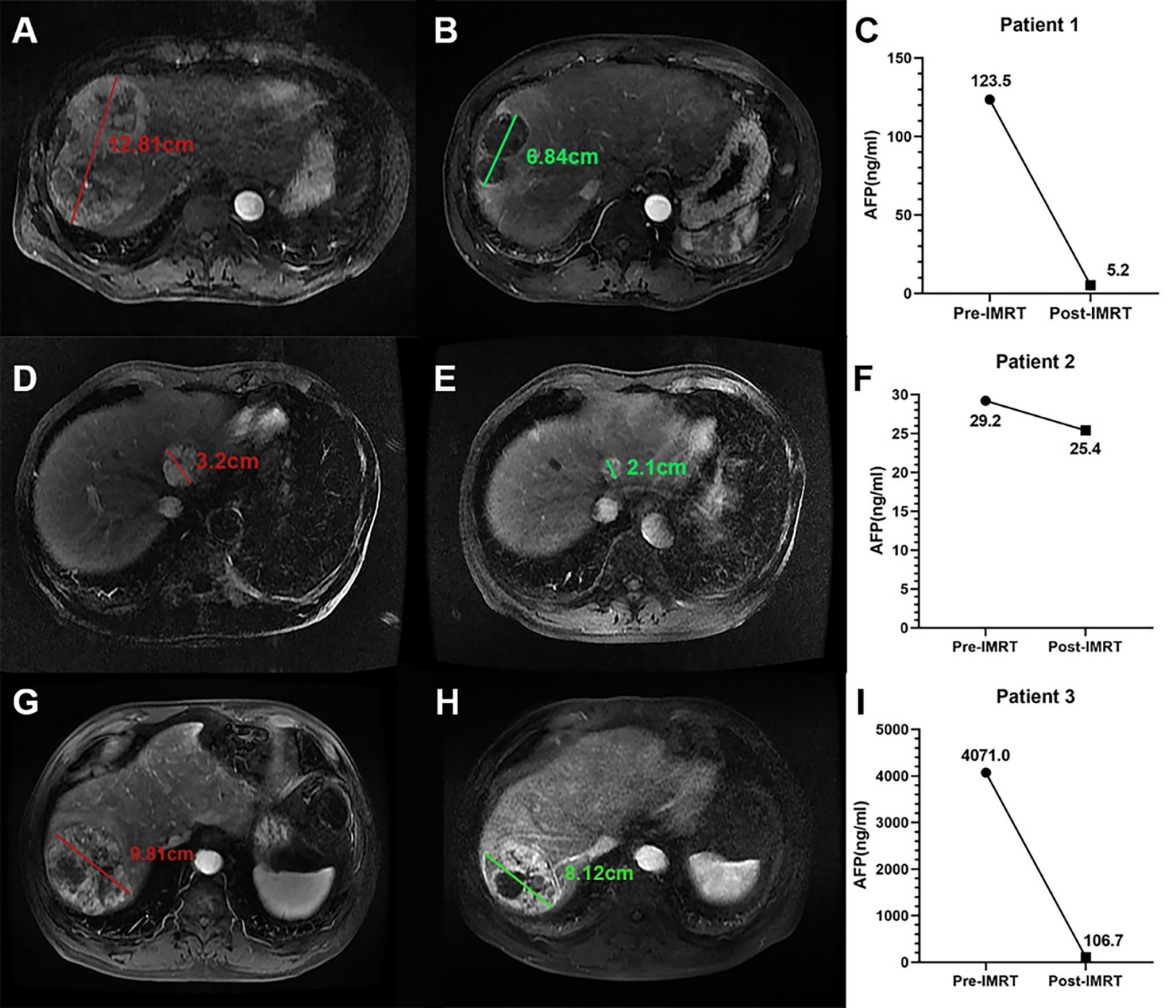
Three example cases of PR defined by the α-RECIST. (A, B) present the SLD on arterial phase images of pre- and post-IMRT CE-MRI scans for patient 1, and the size change is -42.0%; (C) presents the serum AFP levels before and after IMRT for patient 1, and the AFP_Δ_ is -95.8%. (D, E) present the SLD on arterial phase images of pre- and post-IMRT CE-MRI scans for patient 2, and the size change is -34.0%; (F) presents the serum AFP levels before and after IMRT for patient 2, and the AFP_Δ_ is -15.0%. (G, H) present the SLD on arterial phase images of pre- and post-IMRT CE-MRI scans for patient 3, and the size change is -17.0%; (I) presents the serum AFP levels before and after IMRT for patient 3, and the AFP_Δ_ is -97.4%. For patient 1, both the size decrease and the AFP decrease degree exceed the threshold values; For patient 2, only the size decrease degree exceed the threshold value; For patient 3, only the AFP decrease degree exceed the threshold value. All the three patients are defined as PR by α-RECIST.
